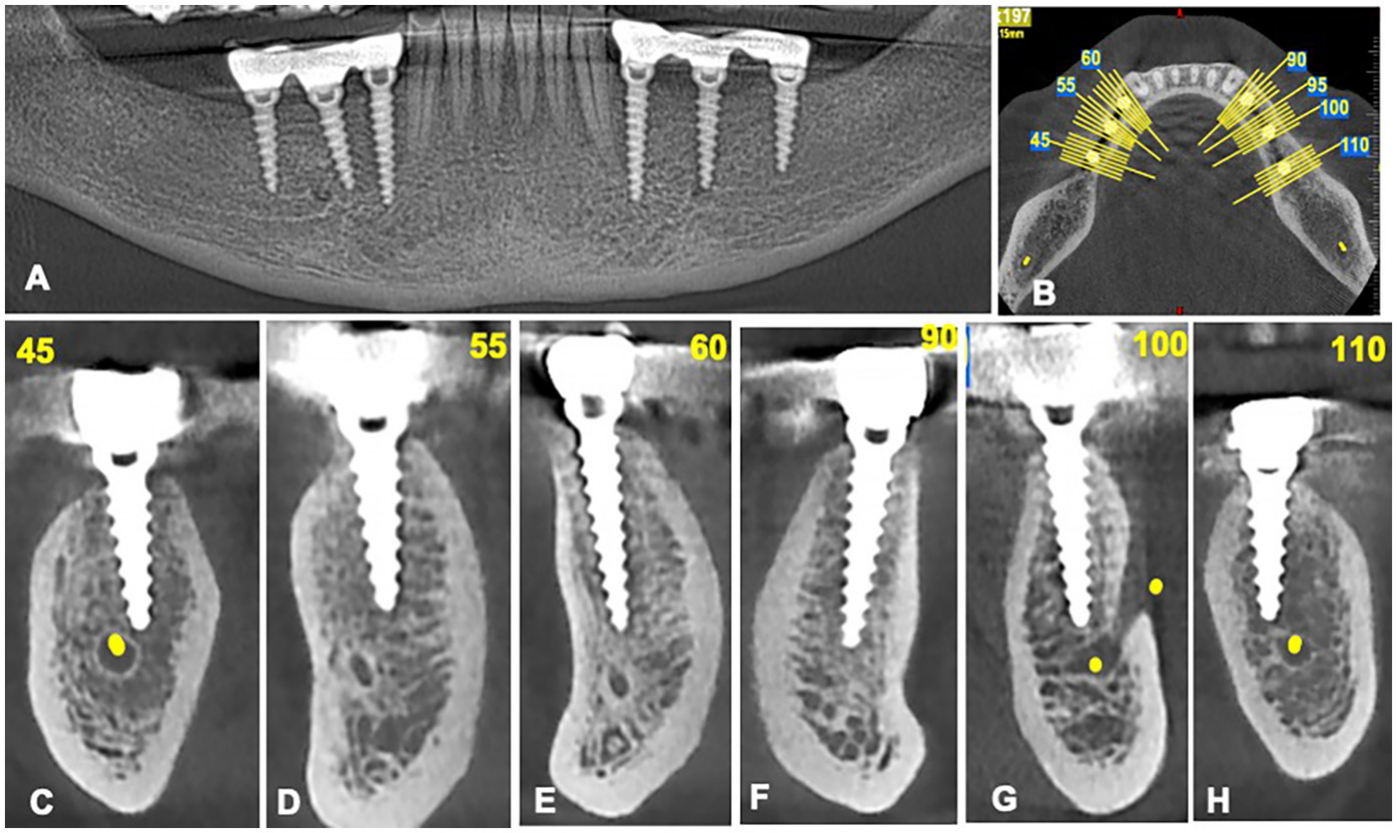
Cone-beam computed tomography images for patients after implant insertion. (A) An overview of the dental implants by orthopantomography. (B) Axial view for the implant placed. (C–H) Sagittal view of dental implants.
